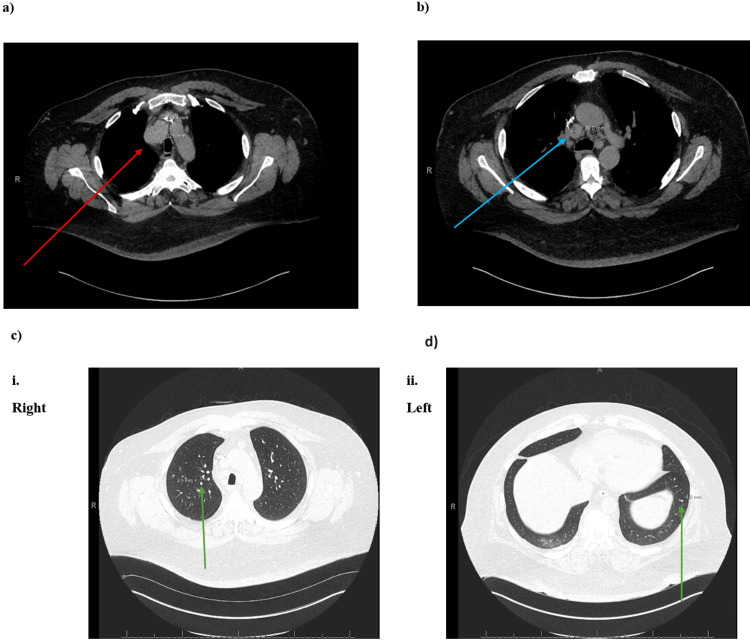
Chest CT Scan Mediastinal Findings: a) Stable mediastinal mass measuring 4.6 x 3.3 cm (indicated by red arrow). b) Stable mediastinal lymphadenopathy measuring up to 1.9 cm in the short axis (indicated by blue arrow). Lungs and Pleura Findings: c) Multiple stable pulmonary nodules throughout both lungs, measuring up to 4 mm. The presence of these nodules had been compared to previous scans, and there was no evidence of new nodules. The largest nodule on each side is indicated by a green arrow, confirming stability and no new areas of concern. Note: The arrows are digitally produced and highlight the areas of interest.
